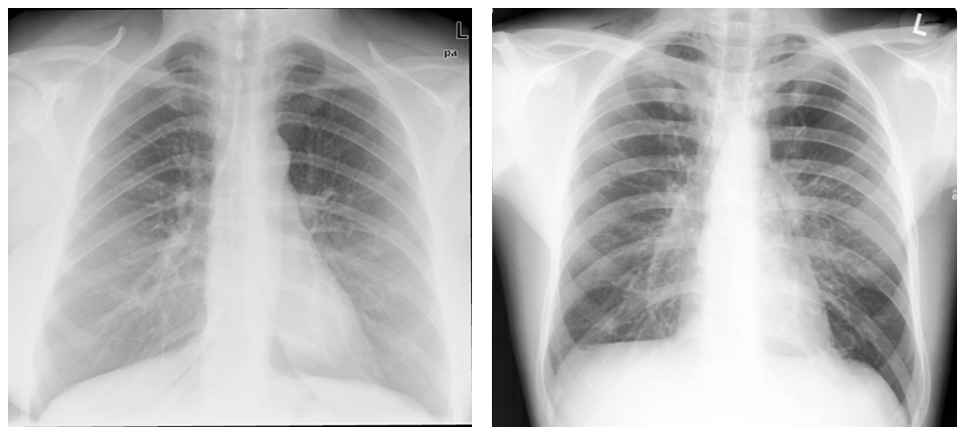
Visually similar medical images contain different semantic meanings. The chest radiographs shown in this figure have a similar visual appearance but belong to different semantic categories. Images are taken from IRMA medical image dataset.
